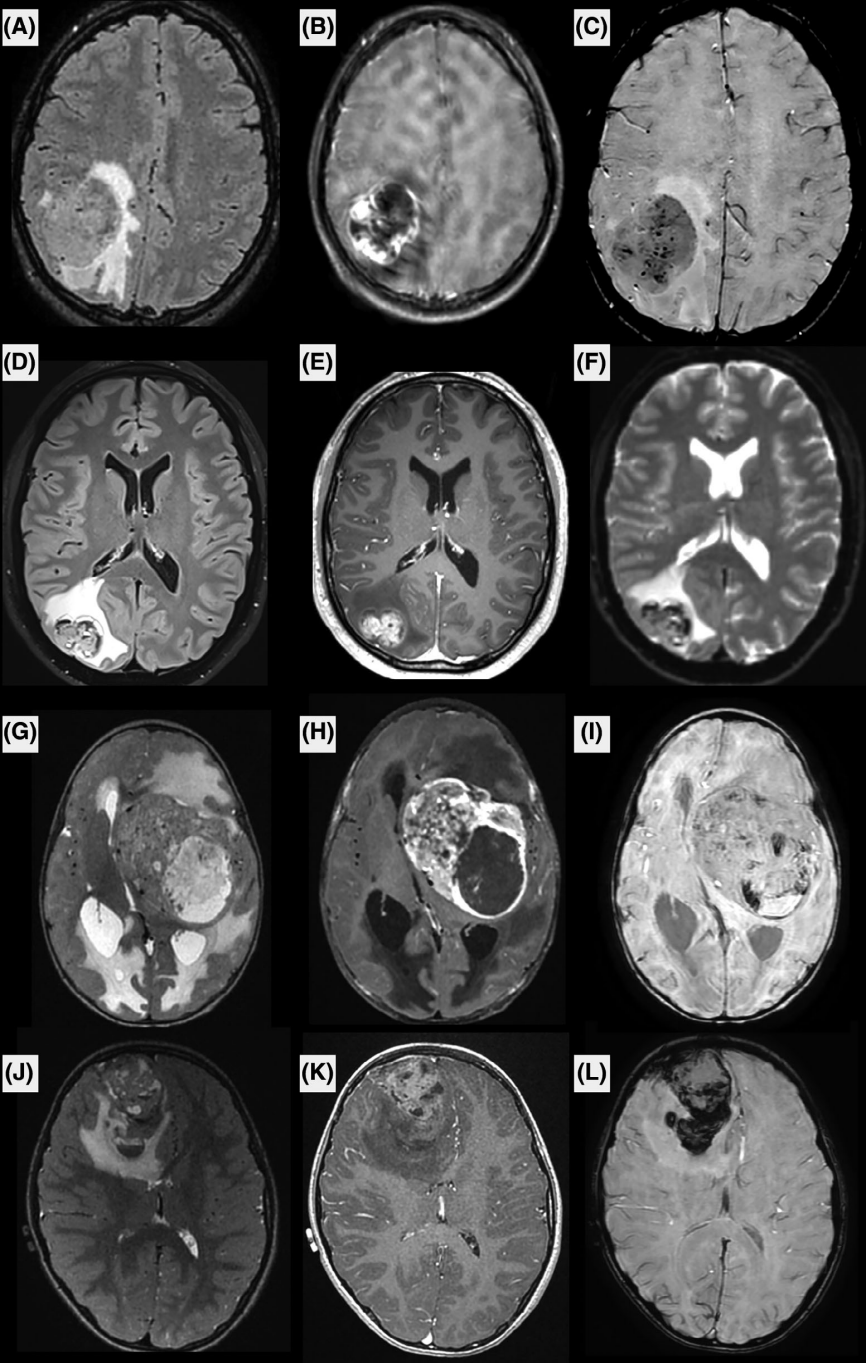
Radiological features of *CIC*‐rearranged sarcomas. Axial FLAIR (A, D), T2‐weighted (G, J), postcontrast T1‐weighted (B, E, H, K), and Susceptibility‐weighted (C, I, L) or echo‐planar (F) images of cases #1 (A, B, C), #3 (D, E, F), #5 (G, H, I) and #6 (J, K, L). The tumors were located supratentorially, and had partially cystic content, intense contrast enhancement, intra‐tumoral hemorrhage, and large peritumoral edema. Most of the tumors had pachymeningeal contact, which is clearly seen in patient #6.
